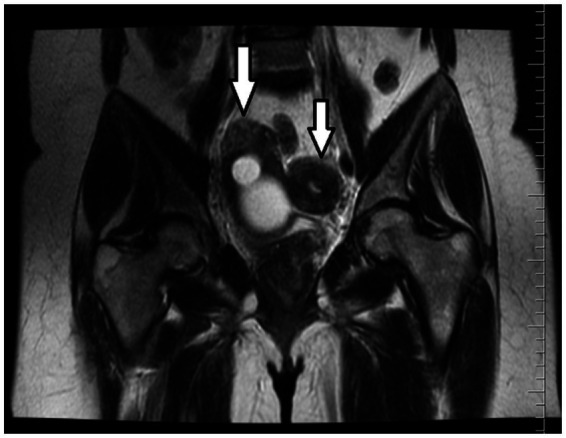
MRI: Coronal T2-weighted image shows the presence of two uterine bodies (white arrows).
